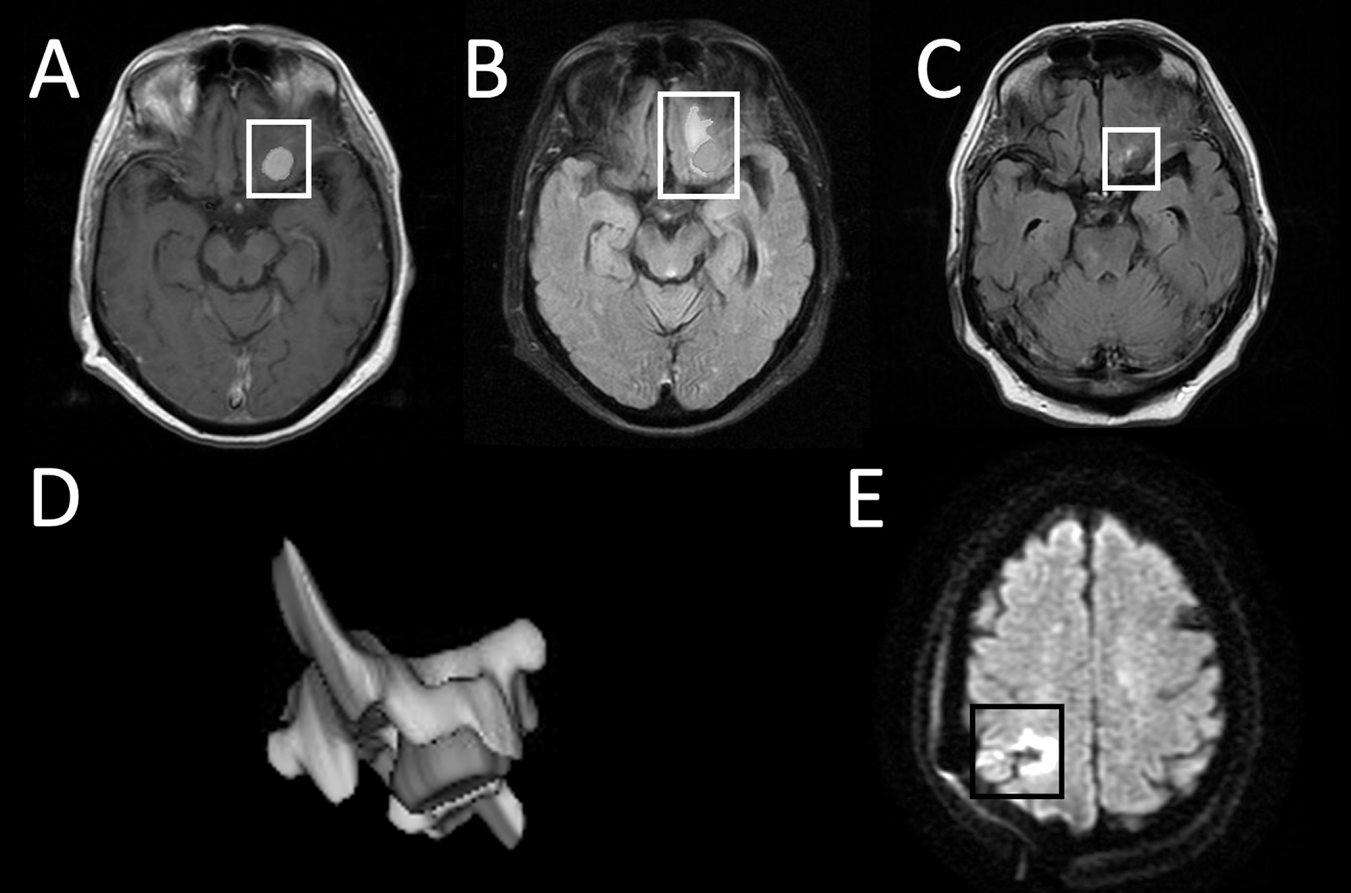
Gadolinium-enhanced T1 images were used for preoperative intracranial meningioma (IM) segmentation. Fluid-attenuated inversion recovery sequence (FLAIR) was used to segment both pre- and postoperative peritumoral brain edema (PTBE). All hyperintensity surrounding the tumor or surgical site was considered as PTBE. (A) T1 + C segmented IM can be seen inside the white box. (B) Segmented preoperative PTBE can be seen in light gray inside the white box. (C) Example of small FLAIR hyperintensity around the surgical site. This small change was classified as persisting PTBE (in the white box). (D) 3D reformat of tumor segmentation in dark gray and surrounding PTBE in light gray. (E) Example of acute ischemia around the surgical cavity in early postoperative in-hospital diffusion weighted MR imaging (in the black box)
